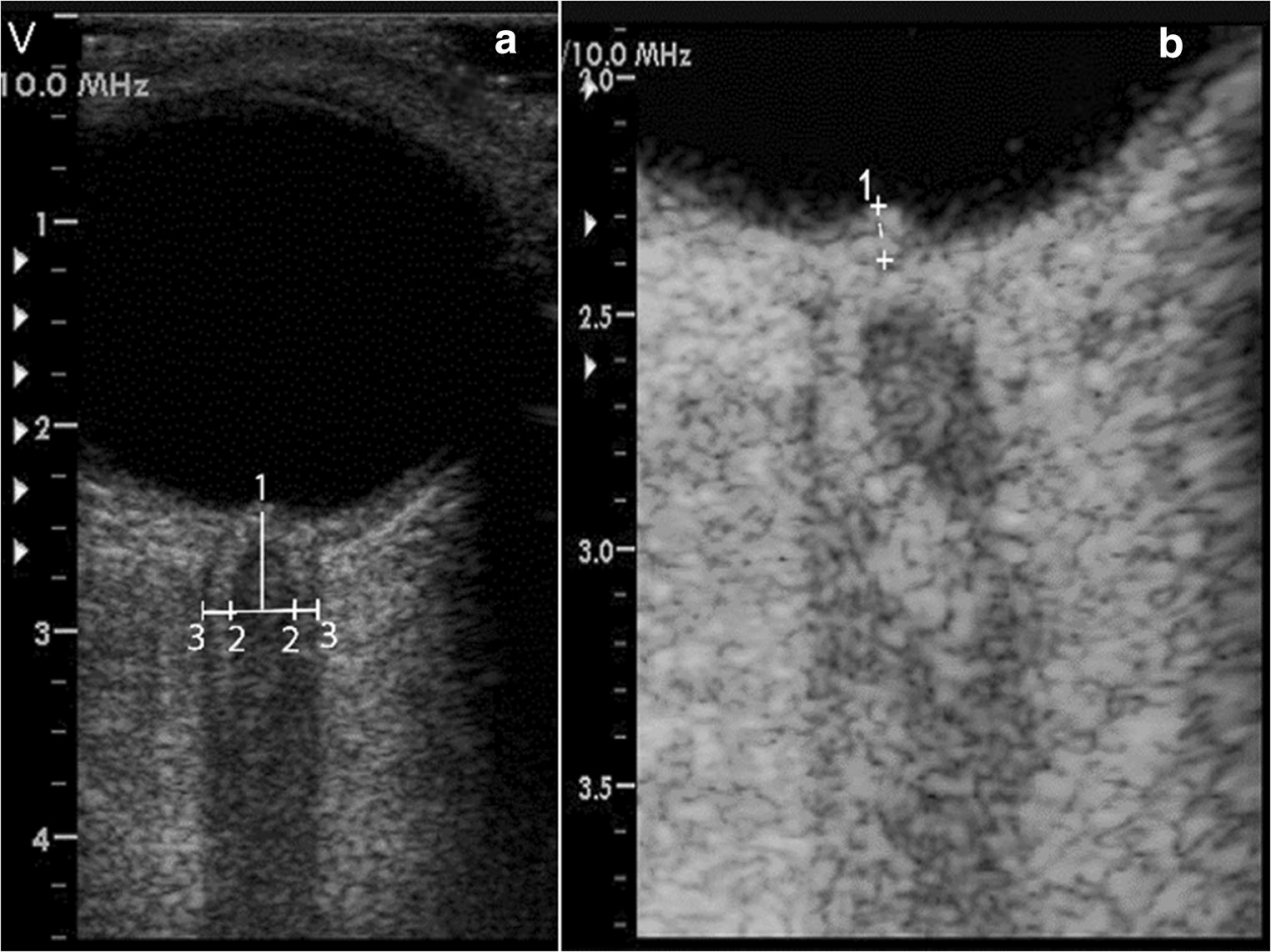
Transorbital sonography showing measurement of the ONSD (distance numbered as 3 while number 2 refers to the optic nerve diameter) performed 3mm behind the globe on the transverse plane (a). Three-times magnification of the optic disc in patient 2 demonstrating elevation of the papilla (b)
